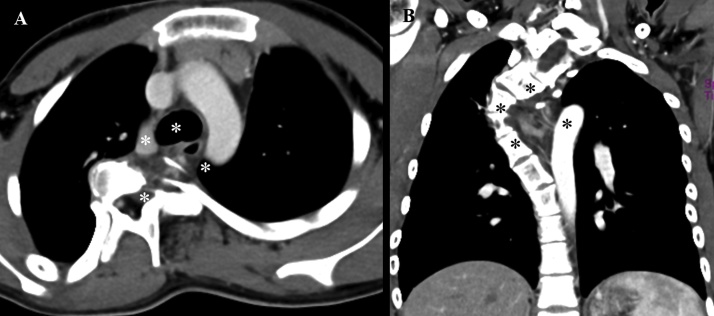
(A) Computed tomography (CT) showing the tumor located in the posterior mediastinum (white asterisks). (B) CT showing scoliosis with the tumor (black asterisks).
